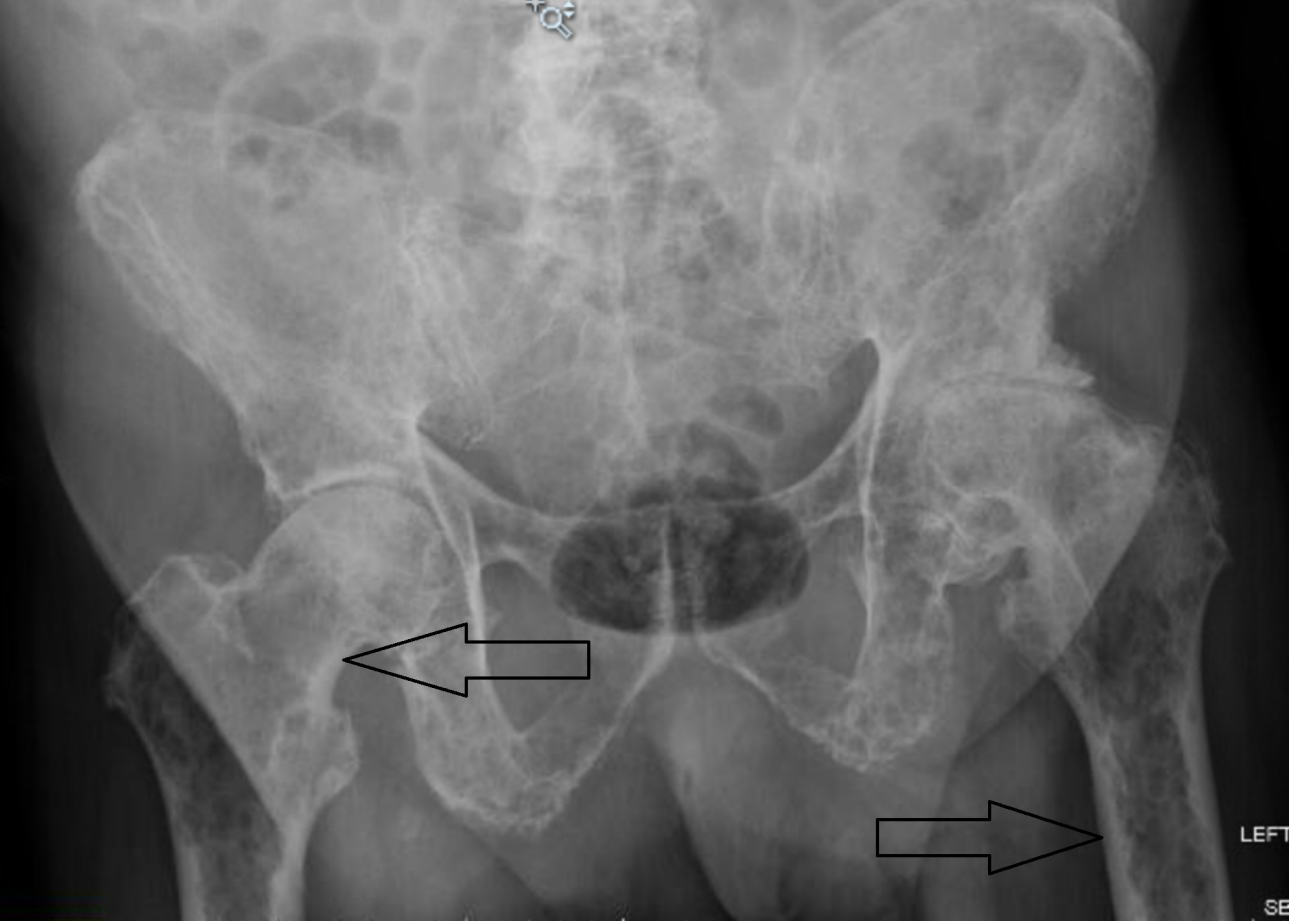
X-ray of the pelvis Showing extensive lytic bone lesions marked by arrows.
